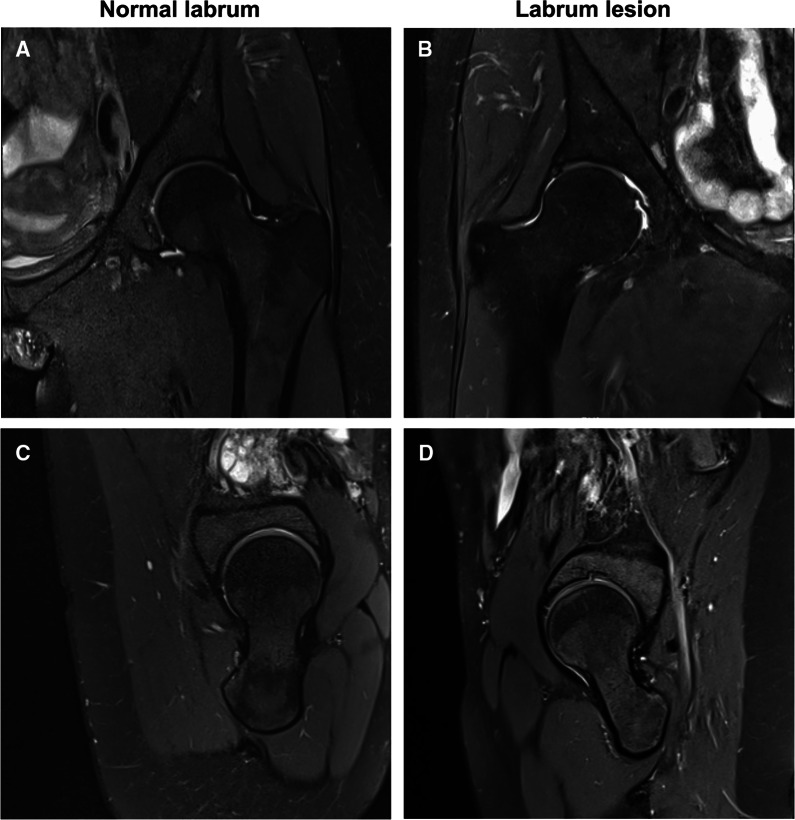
The magnetic resonance imaging of normal labrum and labral lesion. A The normal labrum in oblique coronal plane. B The labral lesion in oblique coronal plane. (C) The normal labrum in oblique sagittal plane. D The labral lesion in oblique sagittal plane
